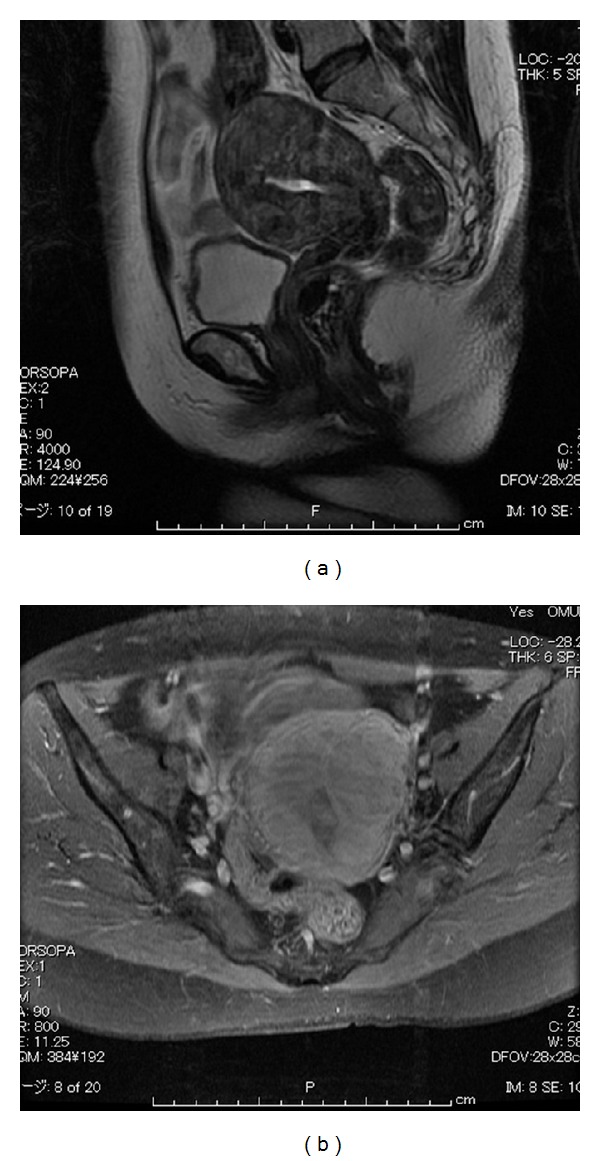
Magnetic resonance imaging revealed multiple myomas. The endometrium was enhanced on T_1_-weighted imaging and endometrial cancer was suspected (b). Myometrial invasion was not evident.
